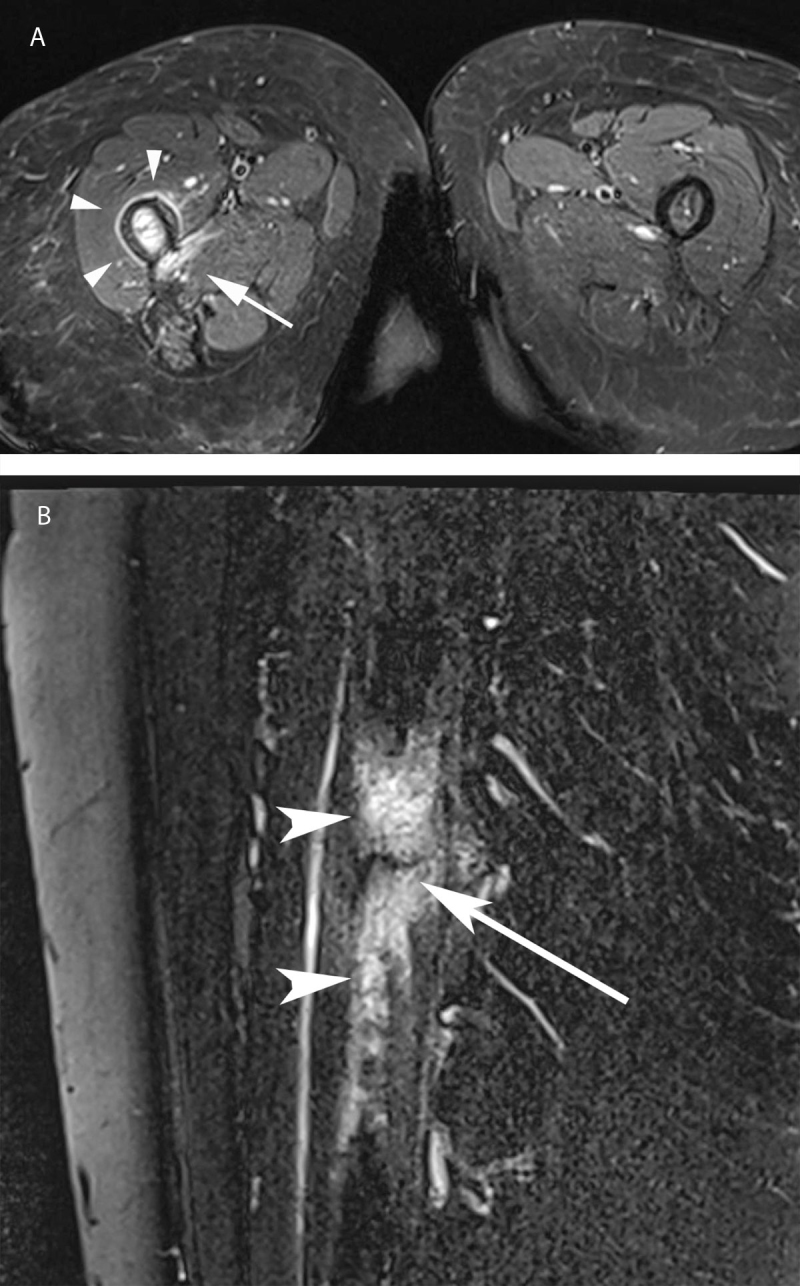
a) Right proximal femur MRI: axial T2-weighted inversion recovery (IR) image shows high signal intensity at the insertion site of right adductors muscles (arrow), extending around the diaphysis as a circumferential ring (arrowheads). b) Right proximal femur MRI: sagittal STIR image shows high signal intensity interesting the posterior soft tissue, and the bone marrow (arrowheads) with a linear low signal component interesting the medial cortical bone of the femoral shaft (arrow), consistent with stress fracture.
